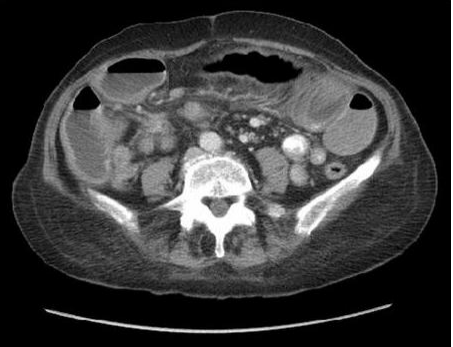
Abdominal CT scan demonstrating dilated loops of bowel, a spiculated lesion in the terminal ileum and mesenteric lymph node enlargement.
